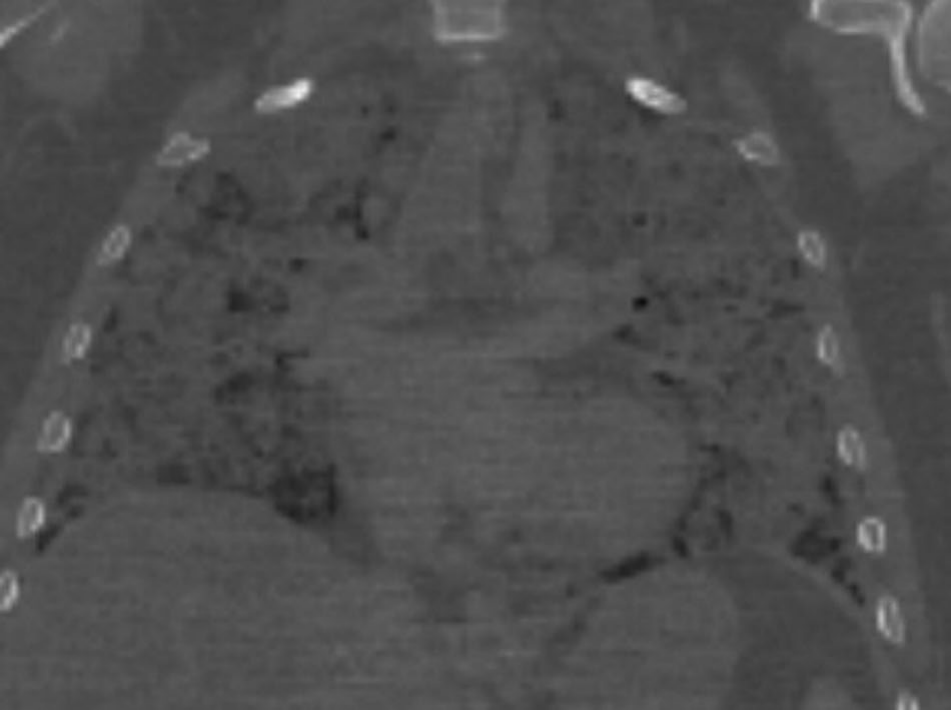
Postmortem CT (PMCT) coronal chest: one 38-year-old male complaining of cough, fever and difficulty breathing for 1 week. He had a negative test for SARS-CoV-2 the week prior to death. There are heterogenous areas of ground glass opacification and areas of consolidation throughout all lobes of both lungs. Although the deceased had a negative test for SARS-CoV-2 prior to death, the PMCT findings with the clinical history was suggestive of COVID-19 and postmortem testing was subsequently positive for SARS-CoV-2.
